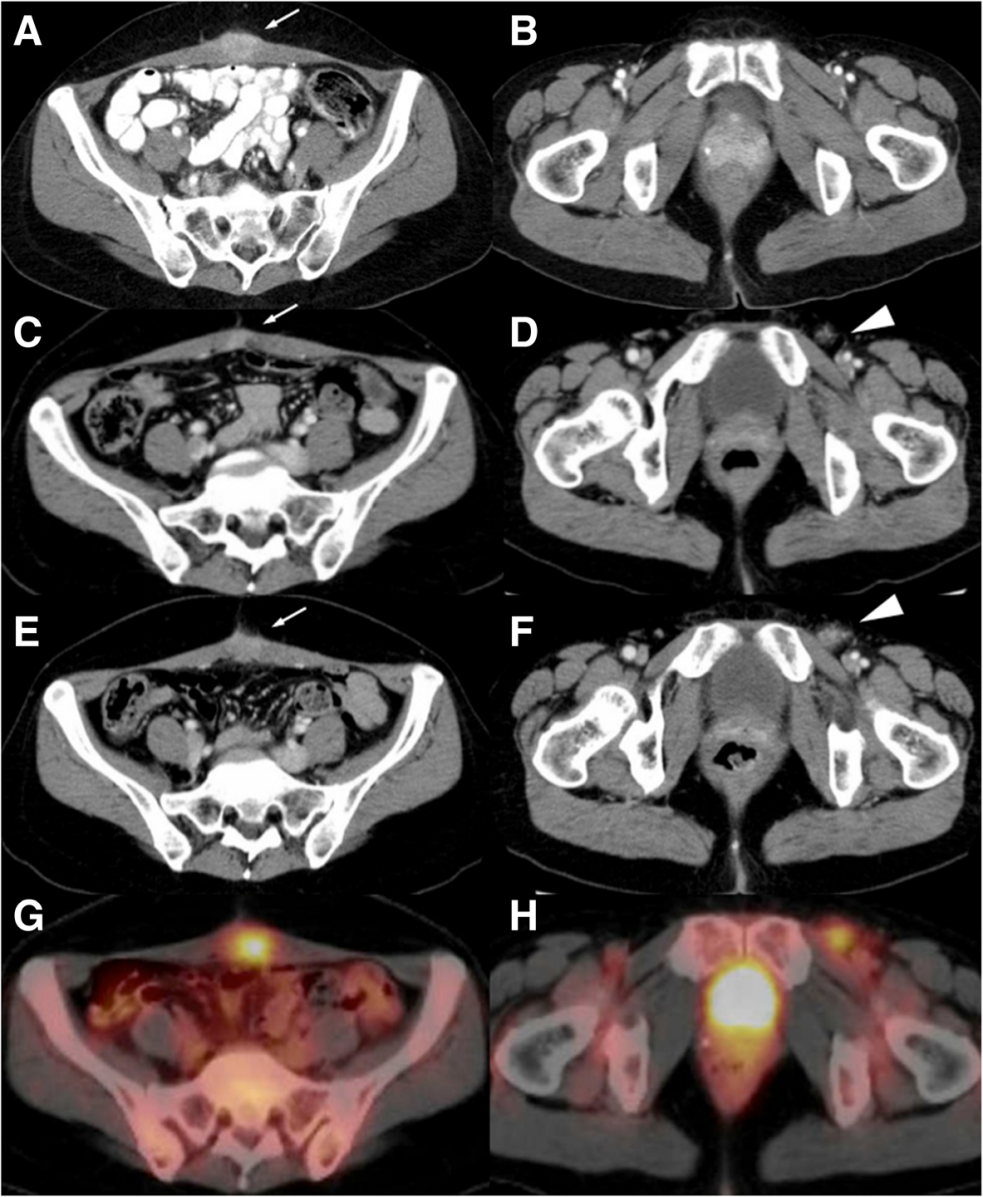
a-b. CT scan before colectomy shows an enhanced mass lesion in abdominal wall (arrow). No enlarged inguinal lymph nodes are evident. c-d. After adjuvant chemotherapy, the mass lesion in abdominal wall decreased in size (arrow). e-f. Two years post-colectomy, CT shows regrowth of a mass lesion (arrow) and enlarged left inguinal lymph nodes (arrow head). g-h. Two years post-colectomy, PET-CT shows significant accumulation of 18F-fluorodeoxy glucose in both lesions
